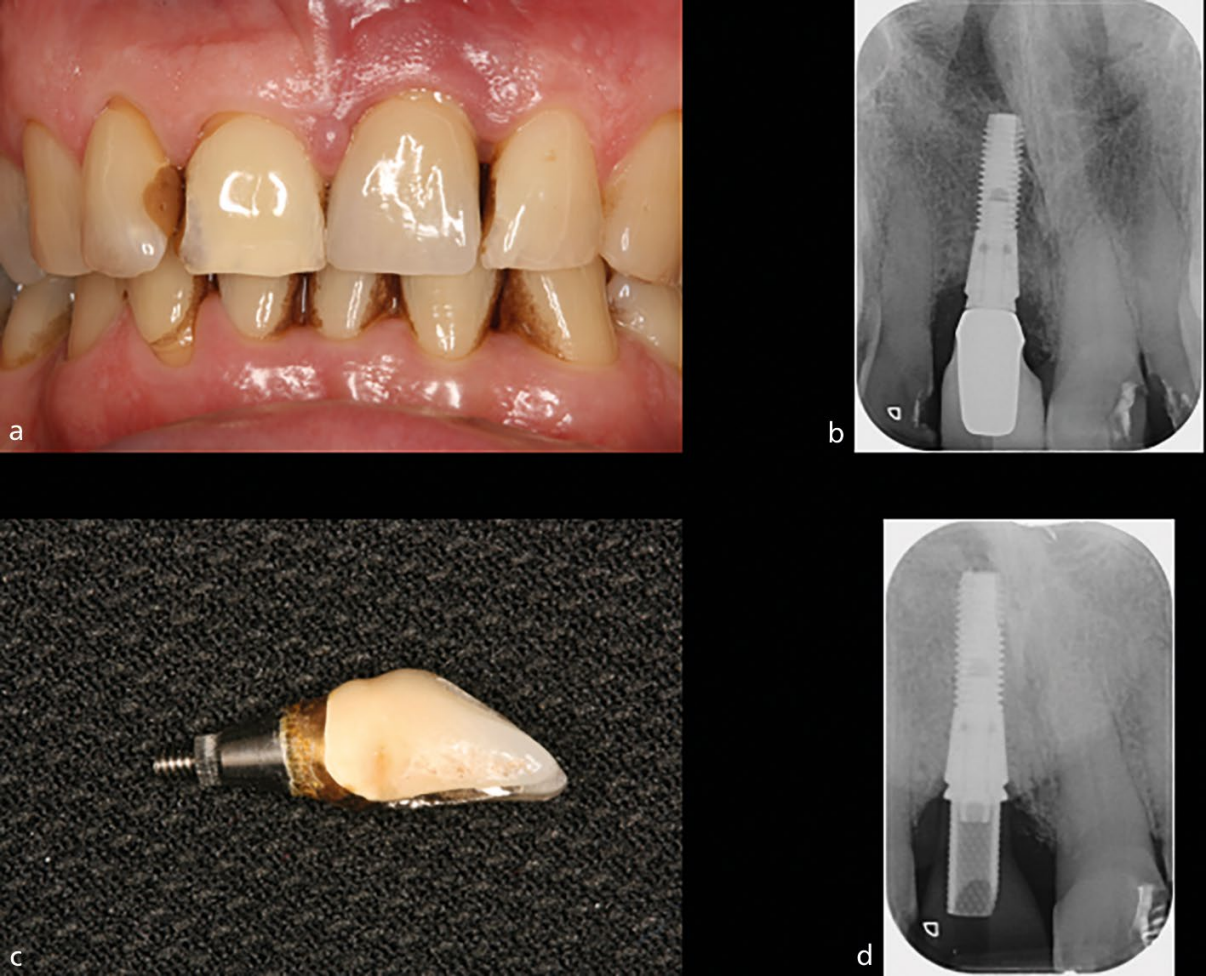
a) This patient presented with pain and suppuration from the labial mucosa of the maxillary right central incisor implant crown. The crown was cement retained and treatment had been provided several years previously. b) The radiograph showed bone loss around the implant head and an incompletely seated crown. c) Access was obtained to the screw and the crown was removed along with the abutment. Excess cement and incomplete seating of the crown was confirmed. d) A well-fitting provisional restoration was provided and bony healing was observed after two years
